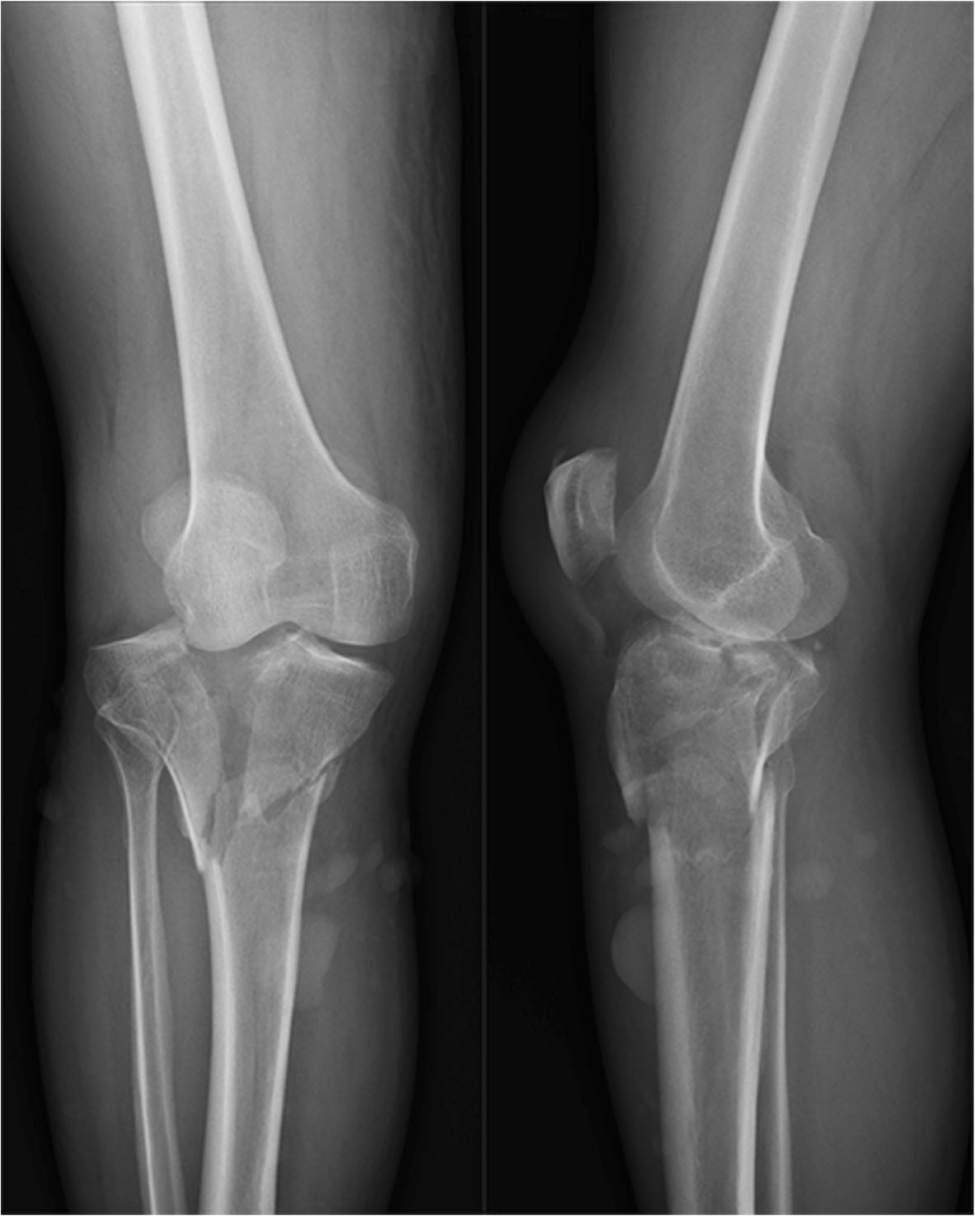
Radiographs of Schatzker type VI fracture, showing widening of the tibial plateau and comminution of the articular surface. The definition of a Schatzker type VI fracture is bilateral plateau fractures associated with metaphysis fracture
